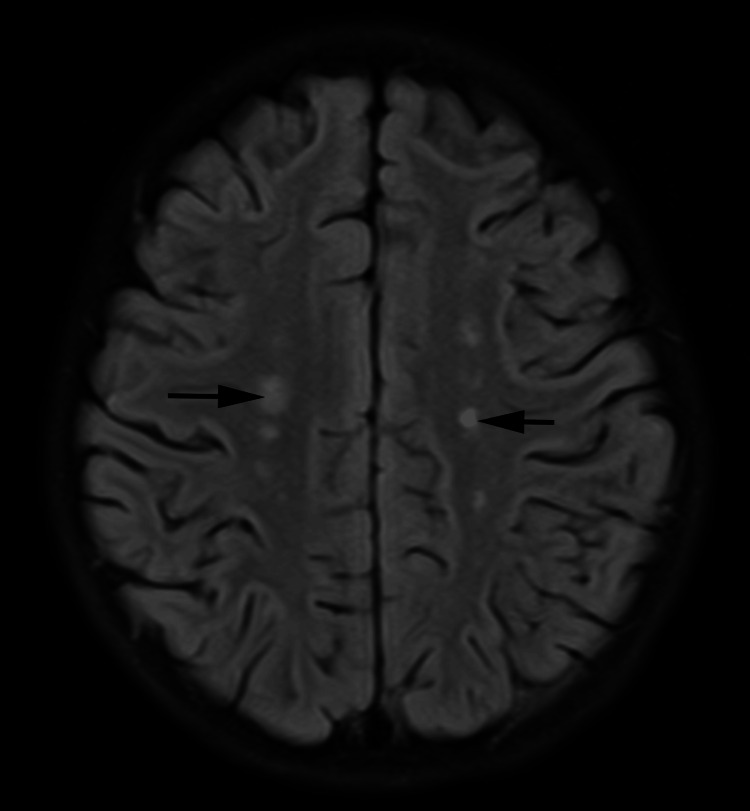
Brain MRI showed multiple abnormal signals in the brain with limited diffusion (black arrow) MRI, magnetic resonance imaging
